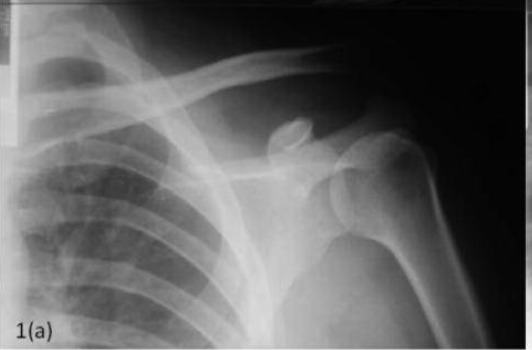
Pre operative radiograph showing superior displacement
